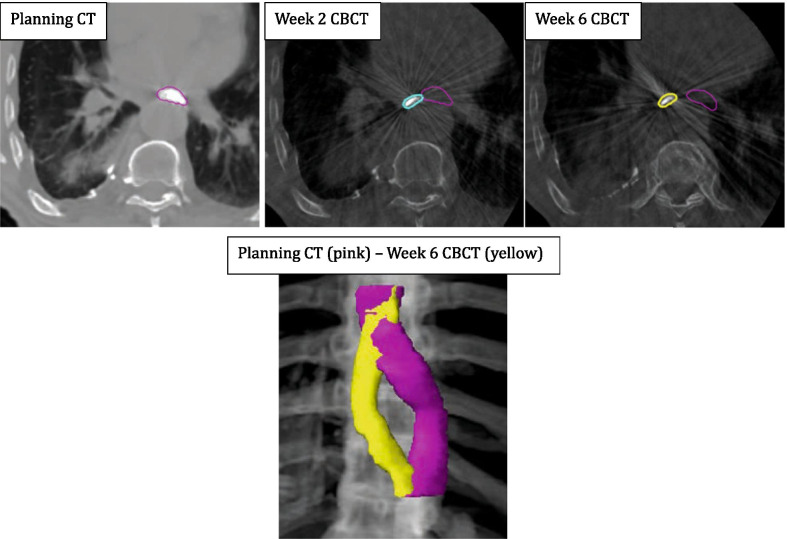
Variation in oesophageal position from planning CT to week 2 CBCT and week 6 CBCT of one patient
